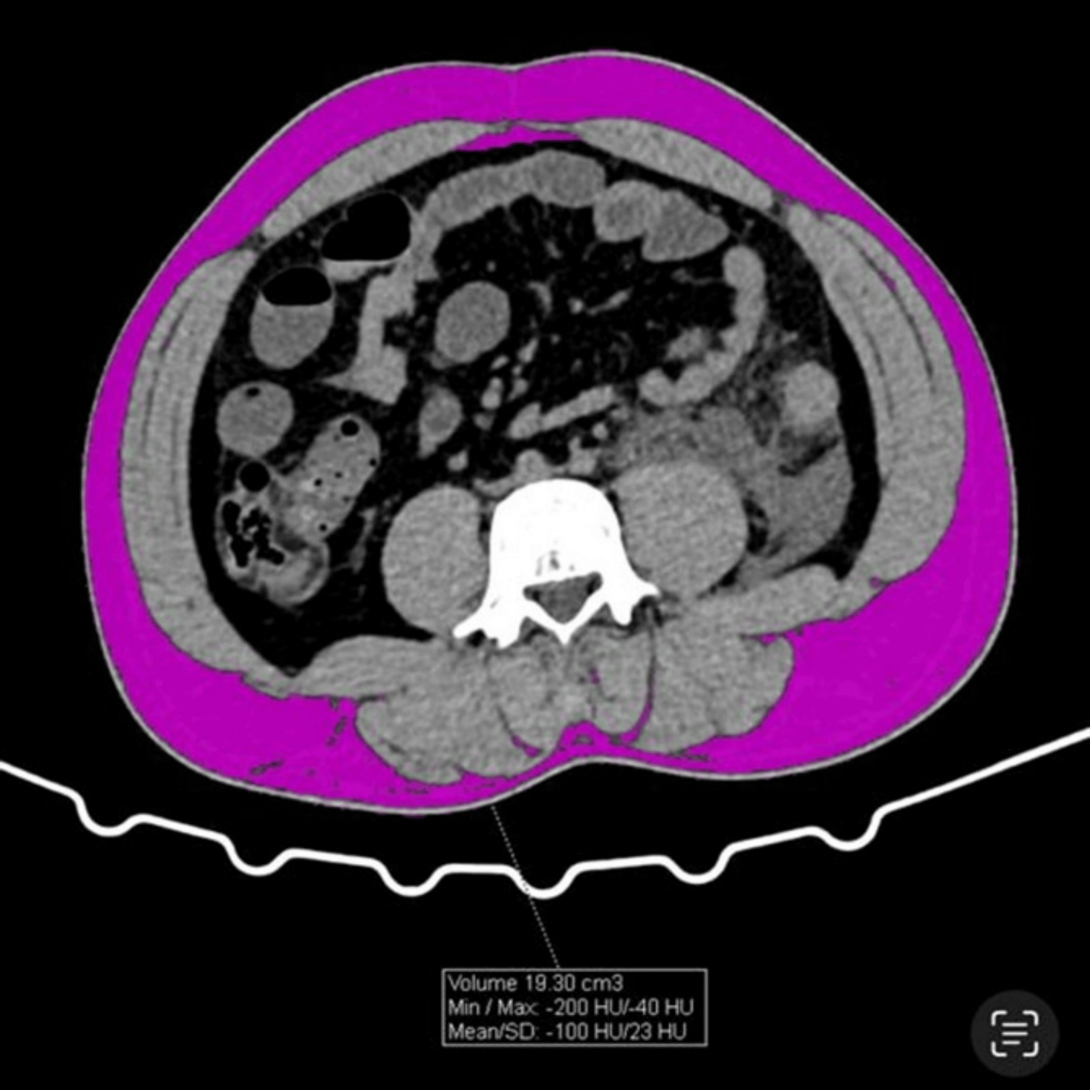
SAT calculations by CT within the ROI (region of interest) SAT: Subcutaneous Adipose tissue
